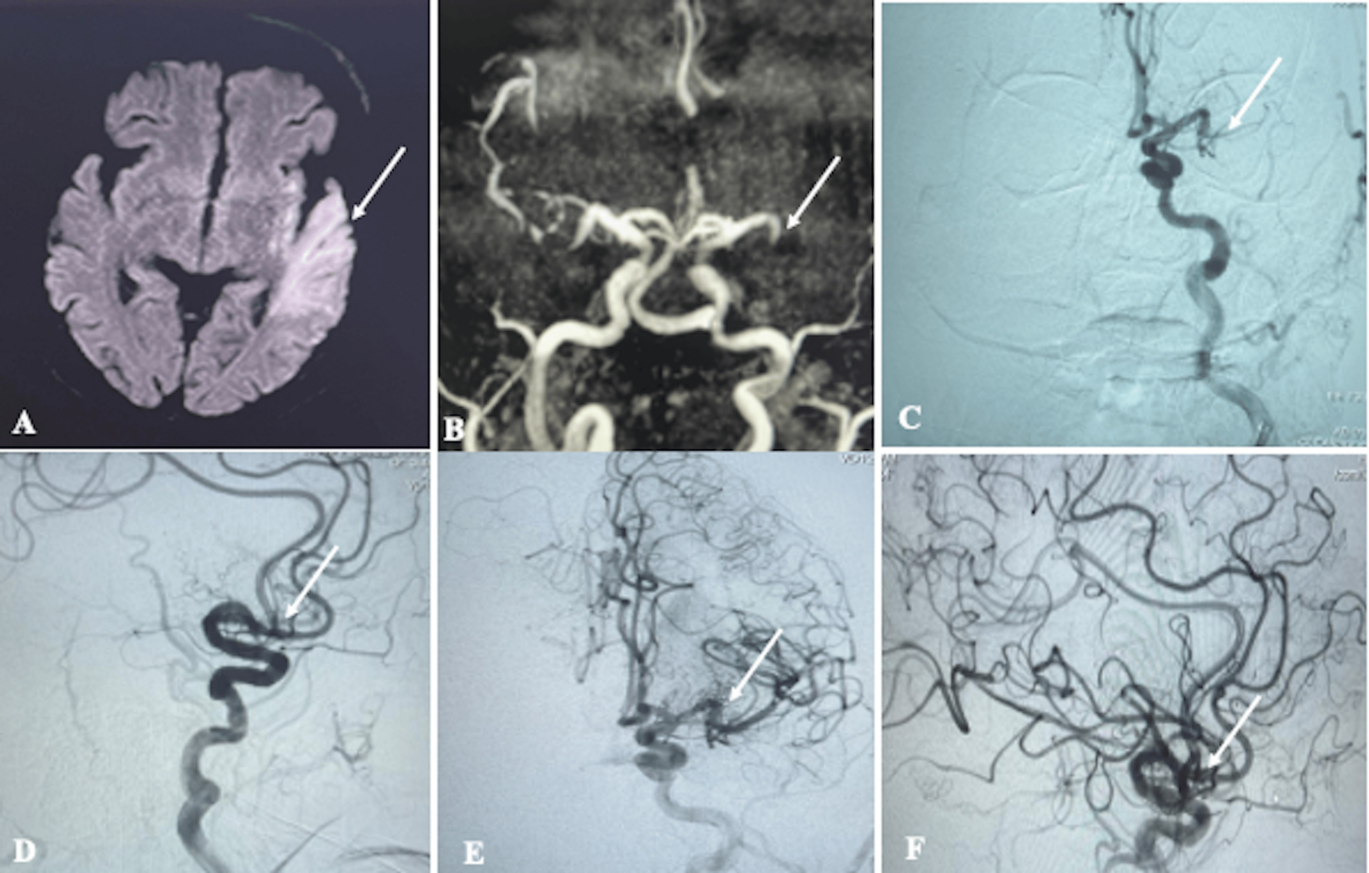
Magnetic resonance imaging (MRI), digital subtraction angiography (DSA) images of the patient with stroke. Axial diffusion-weighted imaging (DWI) (A) showed acute infarct (white arrow) in the left middle cerebral artery (MCA) territory. Time of flight (TOF) image (B) showed left-MCA complete cut-off (white arrow). DSA images (AP in image C, lateral in image D) showed left distal MCA cutoff (white arrows). Post-procedure DSA images (AP in image E, lateral in image F) showed complete recanalization of left distal MCA with filling of the distal branches (white arrows).
